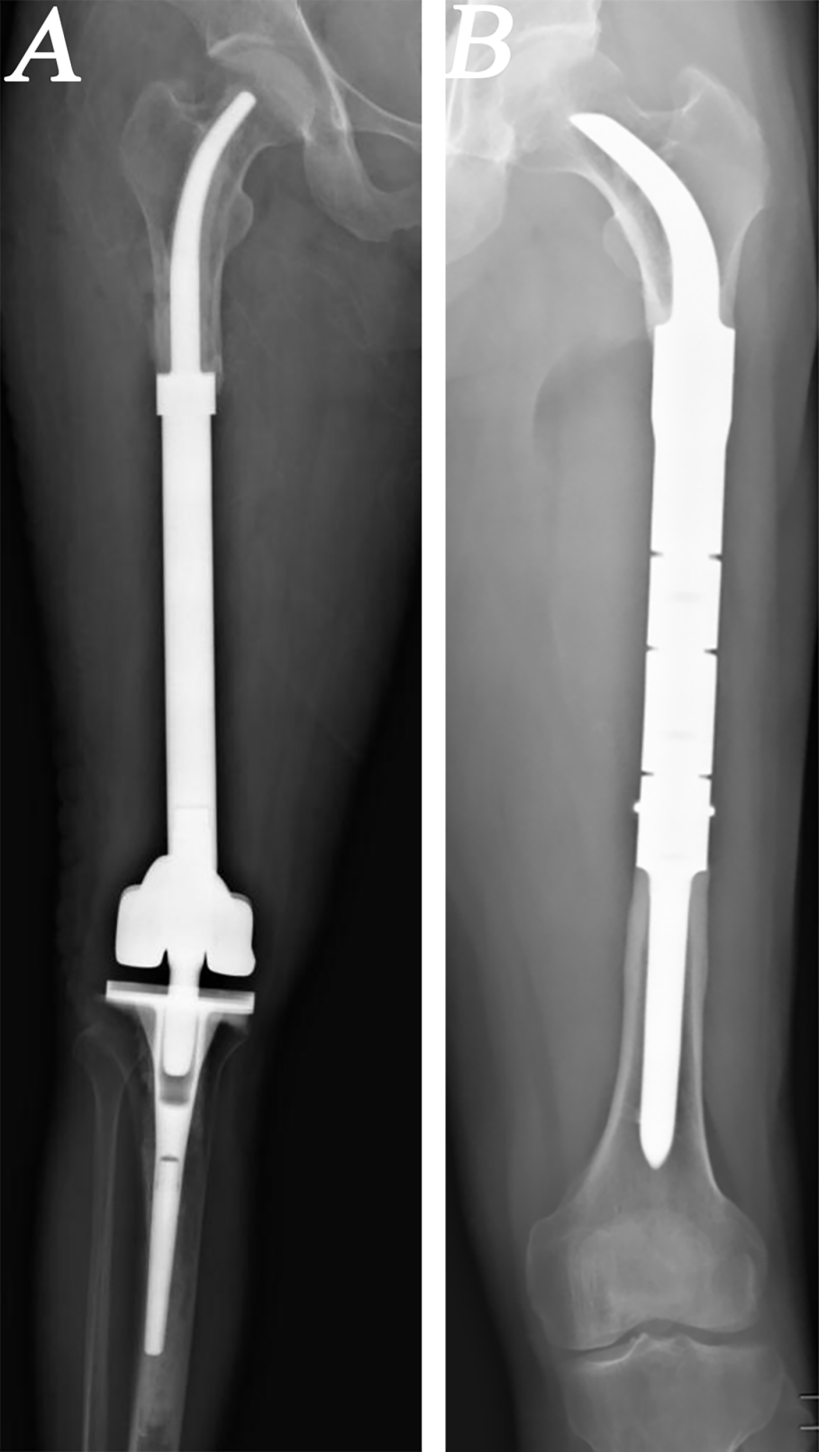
Radiographs of patients who underwent hip-preserving reconstruction (HPR) with intra-neck curved stem (INCS). (A) A patient who underwent HPR with cemented INCS. (B) A patient who underwent HPR with cementless INCS. (HPR: hip-preserving reconstruction; INCS: intra-neck curved stem).
